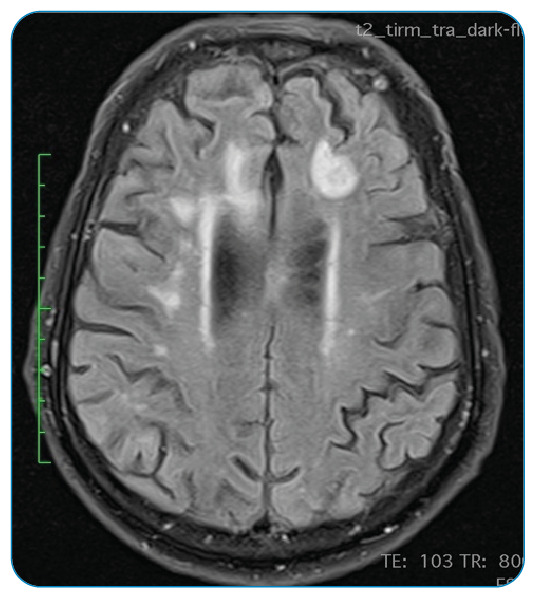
MRI scan on admission. Axial T2-weighted fluid-attenuated inversion recovery. Bilateral frontal subcortical lesional areas with peripheral restriction on difusion and slight vasogenic edema.
